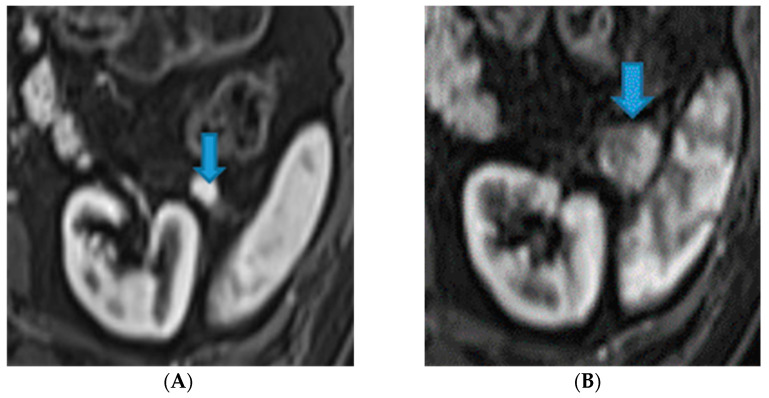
Axial CE portal phase FST1WI. PC from clear cell renal carcinoma: Note the hypervascular deposit adjacent to the spleen that was mistaken for an accessory spleen (arrow in (A)). Observe the growth on the follow-up CT (arrow in (B)).
